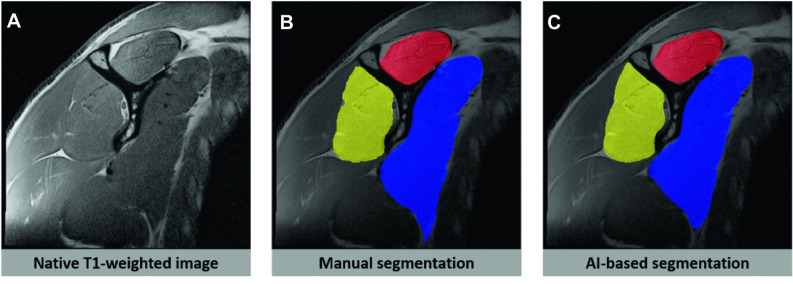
Sagittal T1-weighted MR image of the right shoulder (A), with manual (B) and AI-based (C) segmentations of the subscapularis (blue overlay), supraspinatus (red overlay), and infraspinatus/teres minor (yellow overlay) muscles. The manual (B) and AI-based (C) segmentations had high similarity with a Dice score > 0.93. The segmentation was derived with an AI-based algorithm described by Medina et al. [49]. Images courtesy of Martin Torriani M.D., Harvard Medical School, Massachusetts General Hospital, Boston, MA
